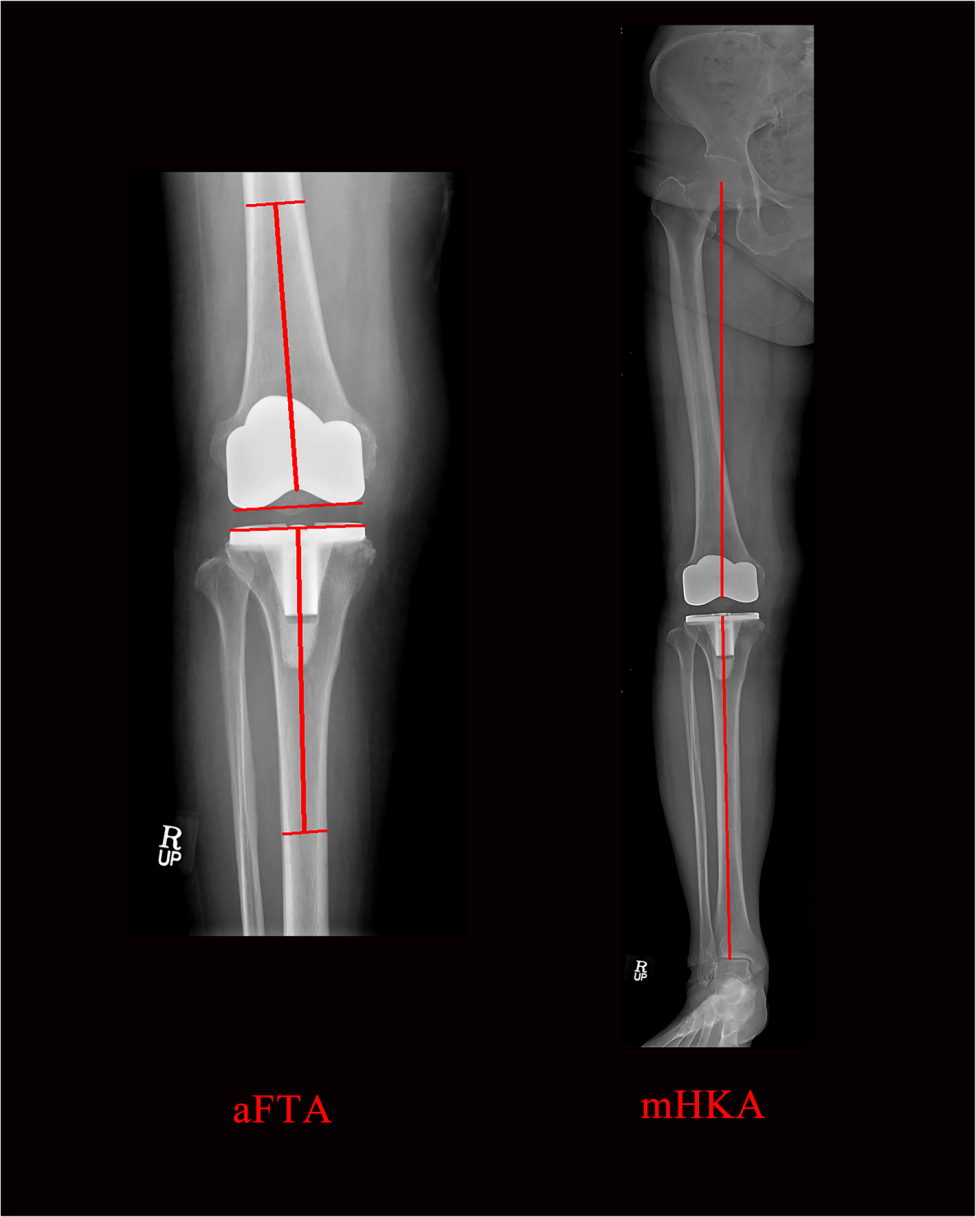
Postoperative short (14 × 17 in. grid cassette) and full-length (14 × 51 in. grid cassette) radiographs showing the measurement of anatomical femorotibial angle (aFTA) and mechanical hip–knee–ankle axis angle (mHKA) The postoperative aFTA was measured using two lines, the first aligned from the bisection point of the femur, 15 cm above the lowermost portion of the femoral component to the deepest part of the center of the femoral component, and the second from the bisecting point of tibia 15 cm down from the uppermost portion of the tibial component [19]. Postoperative mHKA was made between the two lines, the first aligned from the center of the femoral head to the deepest part of the center of the femoral component, and the second from the midpoint of the inner extension of the tibio-talar joint to the center of the tibial component [14].
